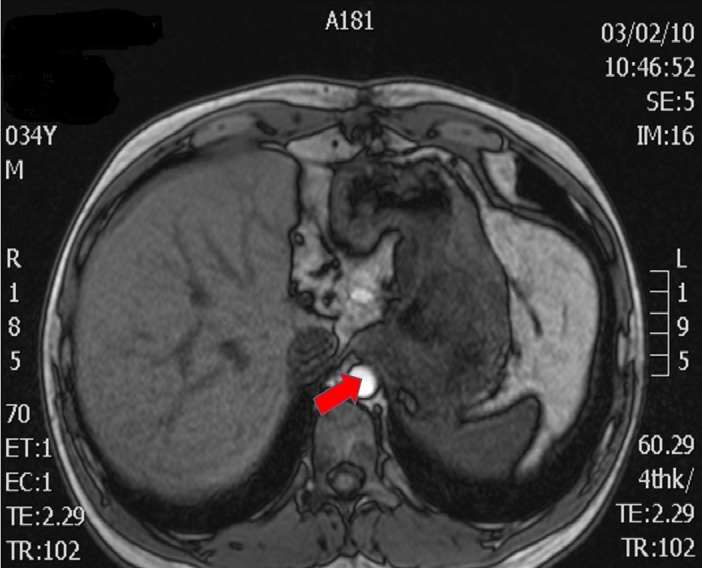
CT and MRI Preoperative Images Axial MRI - Proximity to and potential invasion of descending aorta (arrow)
